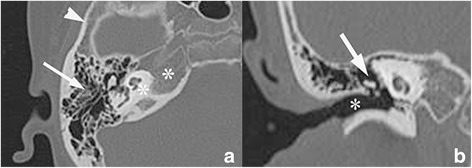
Axial HRCT of the temporal bone shows the five regions used to categorize the lesion (mastoid process: long arrow in a; petrous apex: star in a; squamas: arrowhead in a; external auditory canal: star in b; middle ear: long arrow in b
